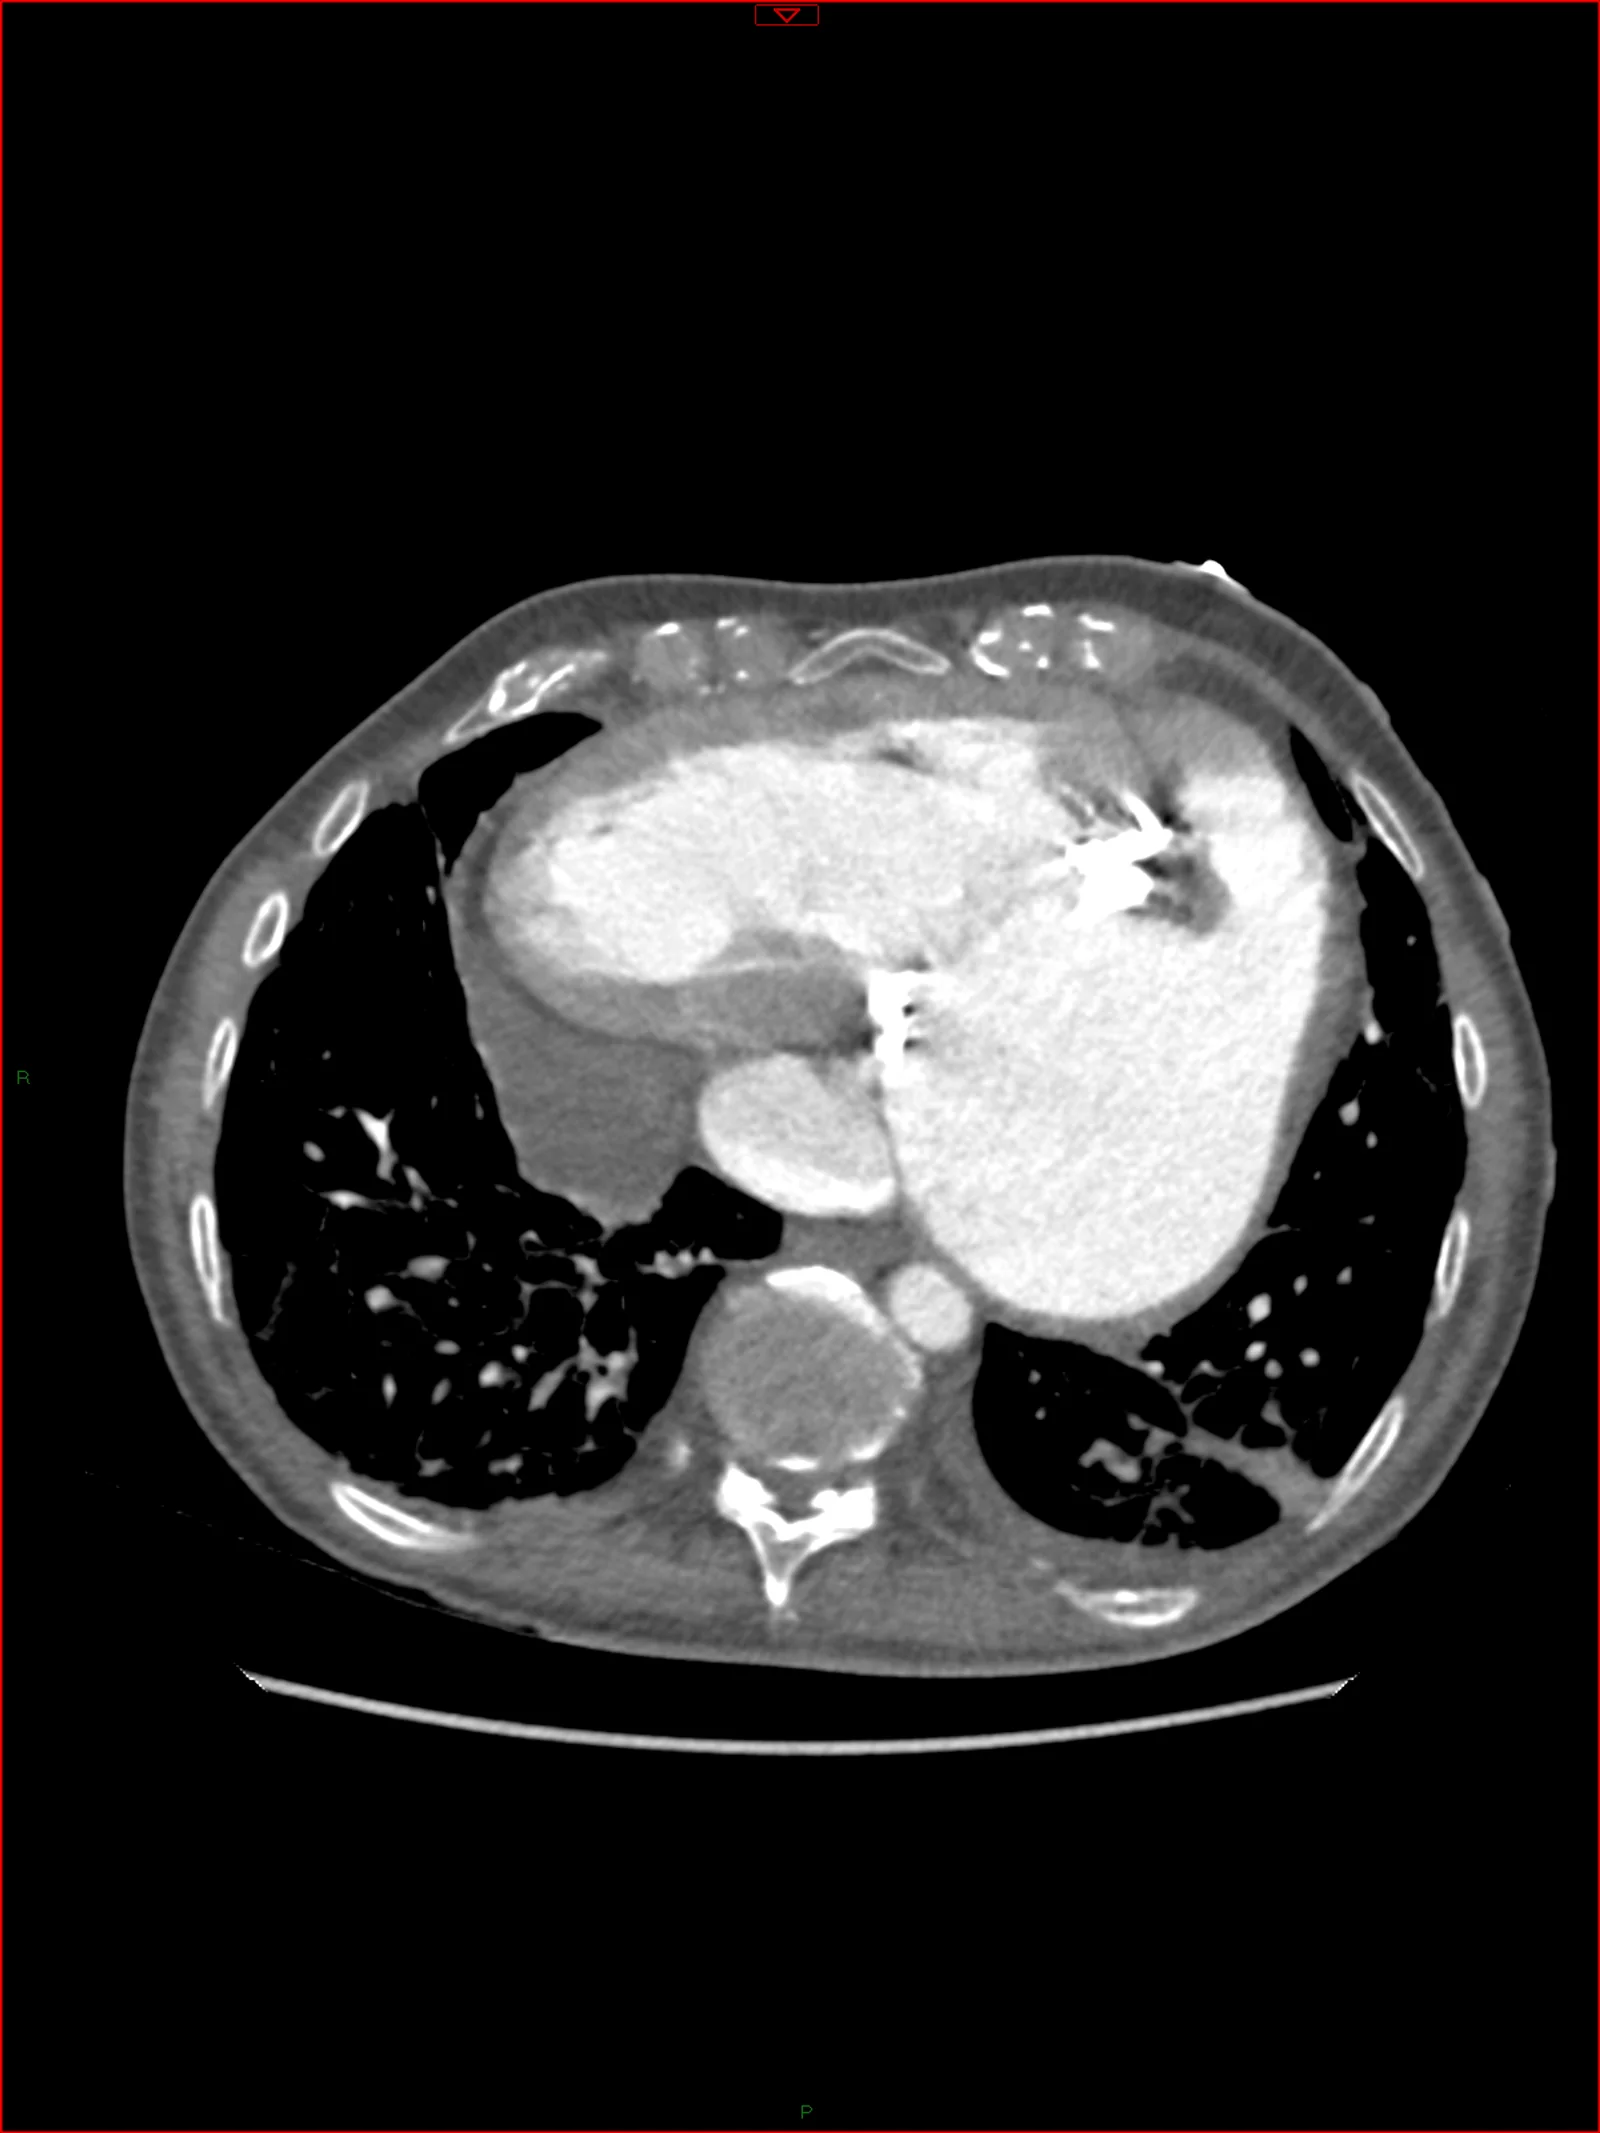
Chest CT angiography, axial image at the ventricular level demonstrating single ventricular chamber CT: computed tomography
